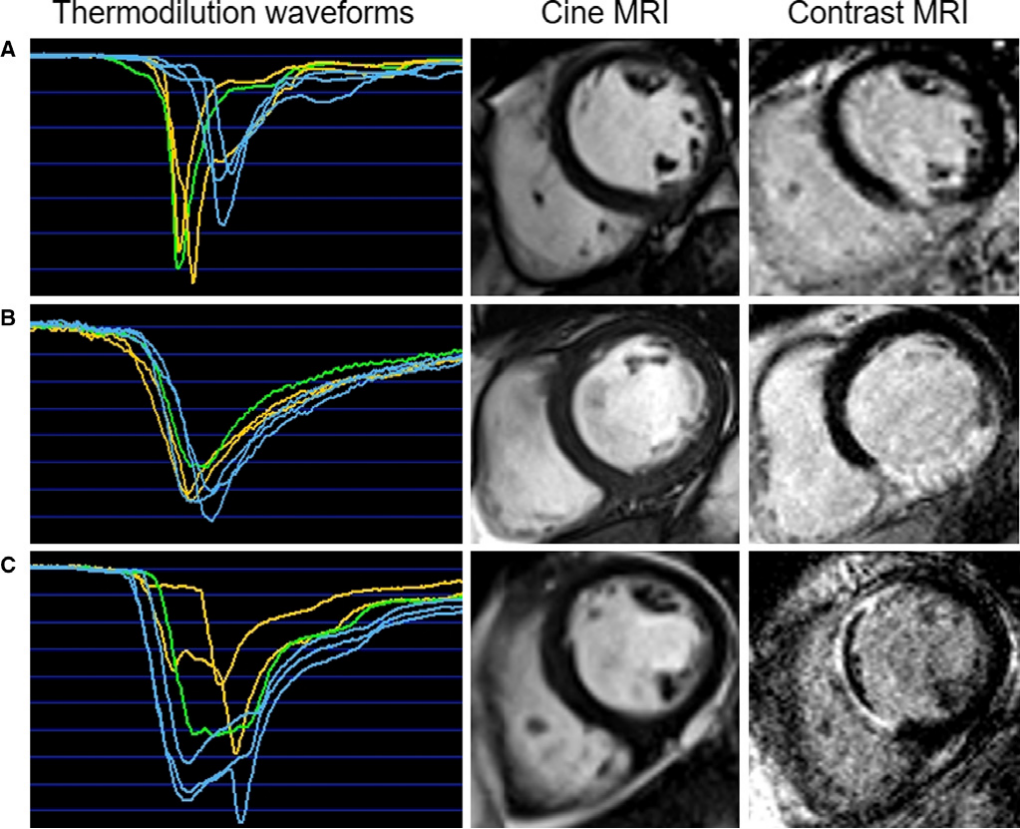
Thermodilution waveforms obtained in the culprit coronary artery at the end of PCI in 3 patients with acute STEMI. The coronary thermodilution curves are obtained in triplicate at rest (blue) and then again during hyperemia (orange) induced by intravenous infusion of adenosine (140 μg/[kg·min]). The green‐highlighted curve represents the “live” measurement of interest according to the software settings. A, A patient with a narrow unimodal waveform and the image obtained using CMR with late gadolinium enhancement (showing a subendocardial infarct with no microvascular obstruction) 2 days later; (B) a wide unimodal waveform and a larger area of transmural infarction revealed by CMR; (C) a bimodal waveform in association with an extensive area of infarction complicated by microvascular obstruction (central dark zone within the bright area of infarction). CMR indicates coronary magnetic resonance imaging; MRI, magnetic resonance imaging; PCI, percutaneous coronary intervention; STEMI, ST‐segment–elevation myocardial infarction.
